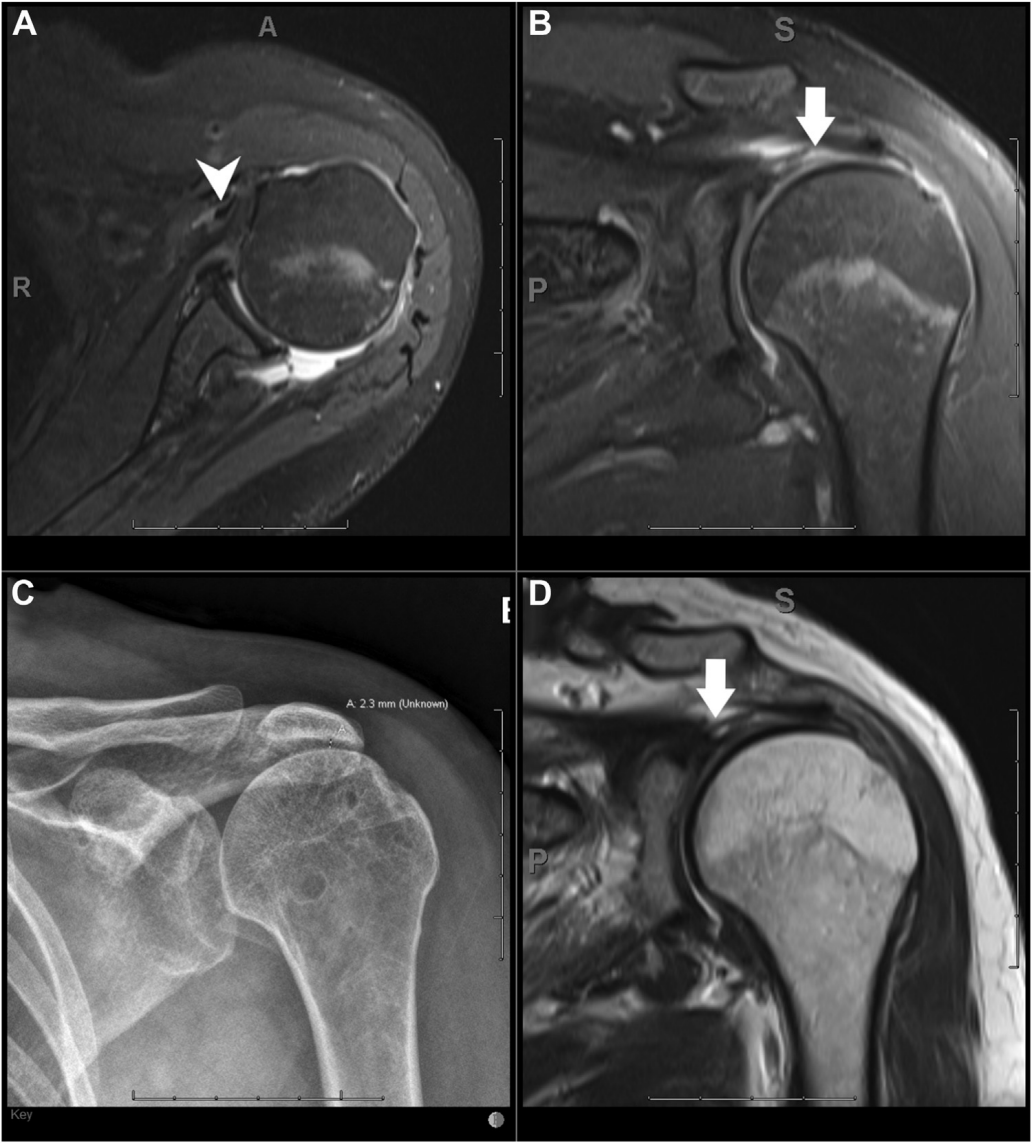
A 3T MRI scan of the left shoulder on a 40-year-old male patient using routine protocol. (A) Axial proton density fat saturated image demonstrating medial dislocation of the PLHB (*white arrow head*) and empty bicipital groove. (B) Coronal proton density fat saturated image and (C) coronal T2 weighted image showing complete full thickness tear of the supraspinatus tendon (*white arrows*). (D) A corresponding frontal radiograph of the left shoulder showing superior humeral head migration with narrowing of the acromiohumeral distance (2.3 mm). *MRI*, magnetic resonance imaging; *PLHB*, proximal long head of the biceps.
